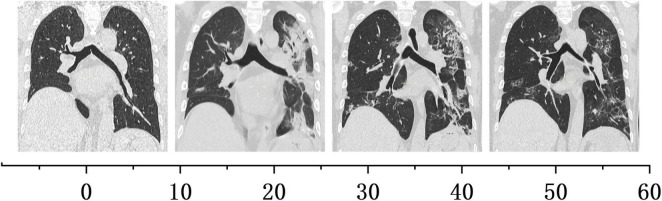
Computed tomography images of lung after kidney transplantation.
